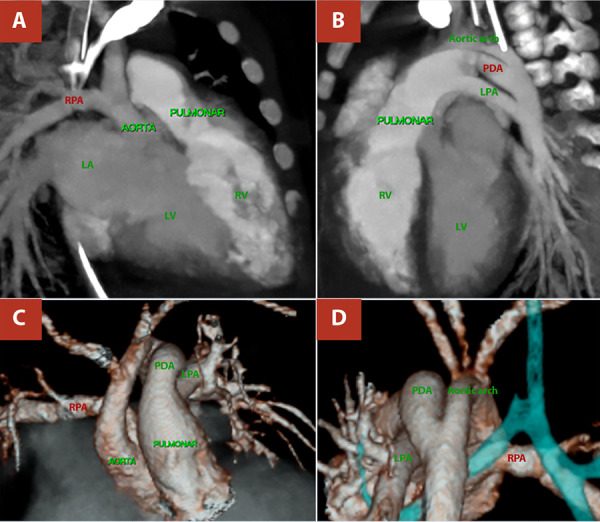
Cardiac and great-vessel computed tomographic angiography, Case 01. A) Anomalous origin of the right pulmonary artery (RPA) from the posterior wall of the ascending aorta. B) Origin of the left pulmonary artery (LPA) from the main pulmonary artery and presence of a patent ductus arteriosus (PDA). C) and D) Three-dimensional reconstruction of the heart and great vessels confirming the findings.
